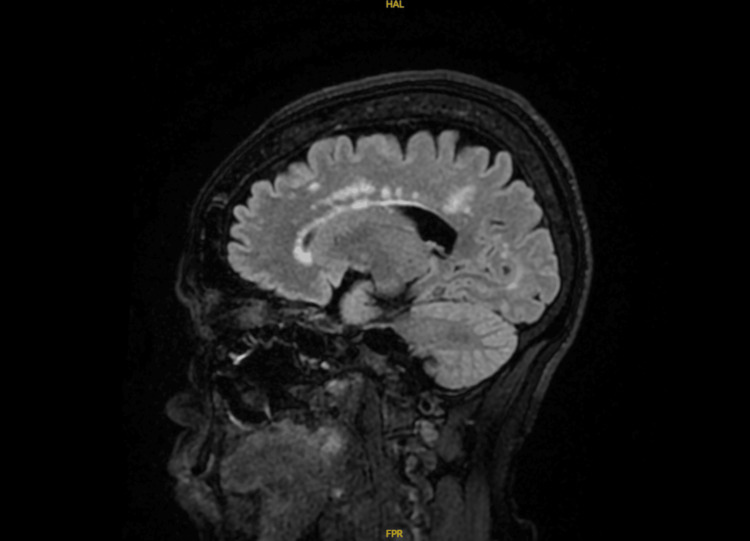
MRI T2 sagittal view showing periventricular white matter lesions.
